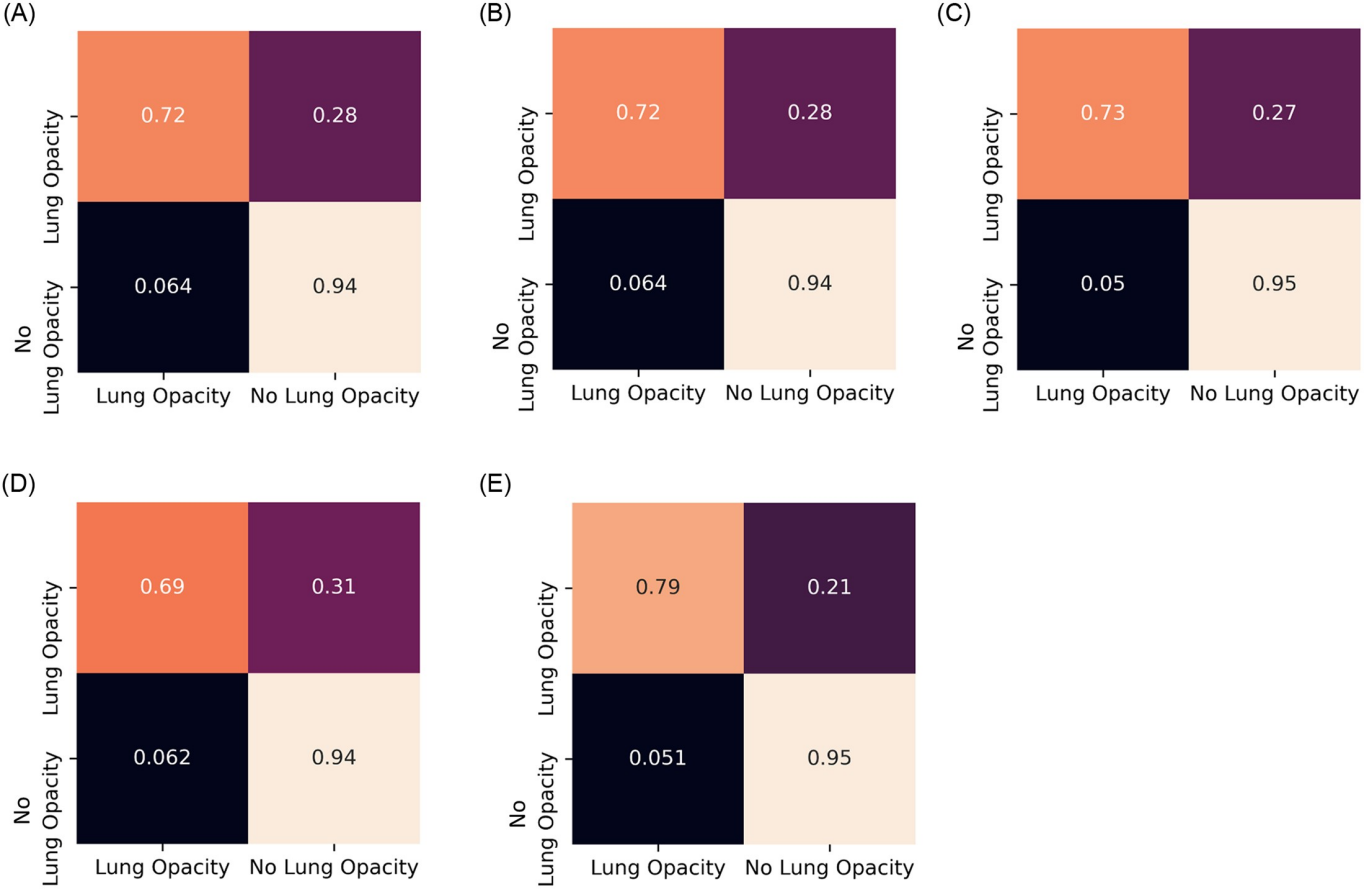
Confusion matrices obtained on the Radiological Society of North America pneumonia challenge chest X-ray dataset by the proposed method by five-fold cross validation. a) Fold-1. (b) Fold-2. (c) Fold-3. (d) Fold-4. (e) Fold-5.
